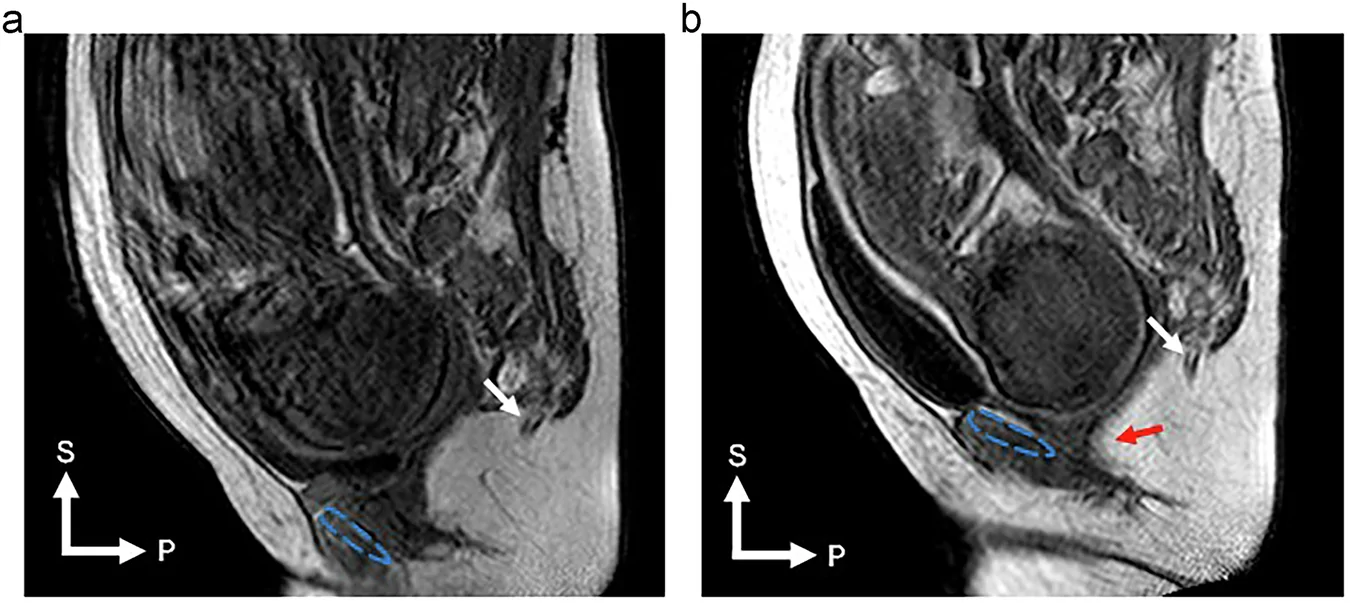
Sagittal MRI before (a), during the SSL (b). Fatty tissue (red arrow) between the vagina (not visible) and the sacrotuberous ligament (white arrows) shifted to the paramedian sagittal plane in all 7 patients during the SSL. The local fat moved to fill zones of weaker resistance inferiorly and para-medially, with the greatest movement being observed in the region of the anal sphincter. The net result of these changes was perineal bulging. The blue dotted line traces the pubic symphysis. MRI, Magnetic resonance imaging; SSL, Second stage of labor
